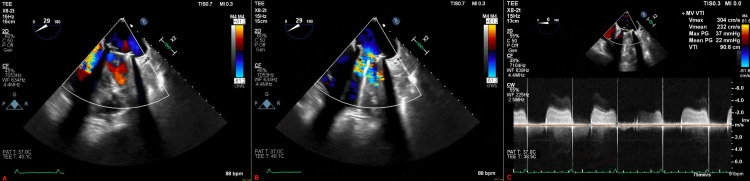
Initial transesophageal echocardiogram. (A) Mechanical prosthesis during systole, (B) mechanical valve prosthesis during diastole with turbulent blood flow on color doppler, and (C) elevated pressure gradients across the mechanical prosthesis.
